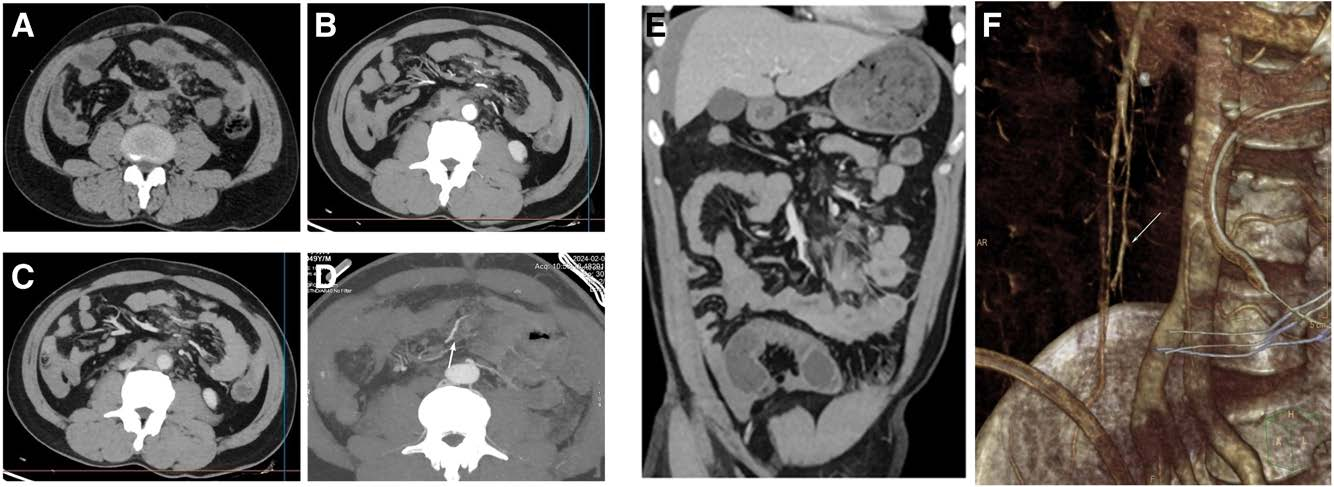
(A–C) Axial CT plain scan, enhanced CT arterial phase, and portal vein phase, respectively. (D) The maximum intensity projection of mesenteric artery CTA. (E) The portal phase of coronal CT enhancement. (F) Mesenteric artery CTA volume reconstruction. CT = computed tomography, CTA = computed tomography angiography.
